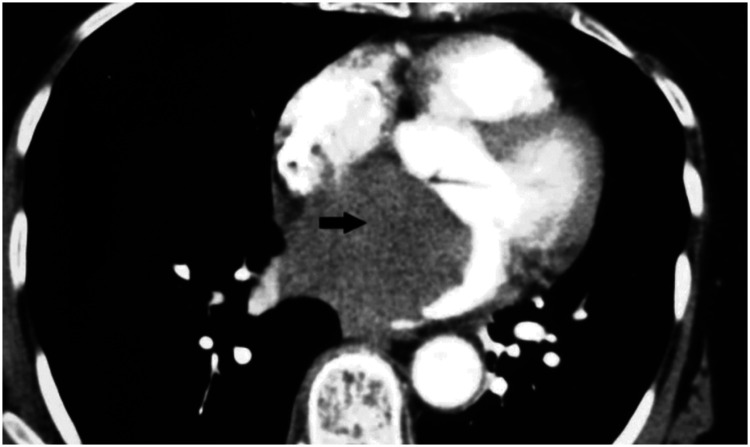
Cardiac computed tomography image shows a large, homogeneous, non-contrast-enhanced mass with low attenuation at the right atrium, suggesting cardiac angiosarcoma (black arrow) (used with permission).
